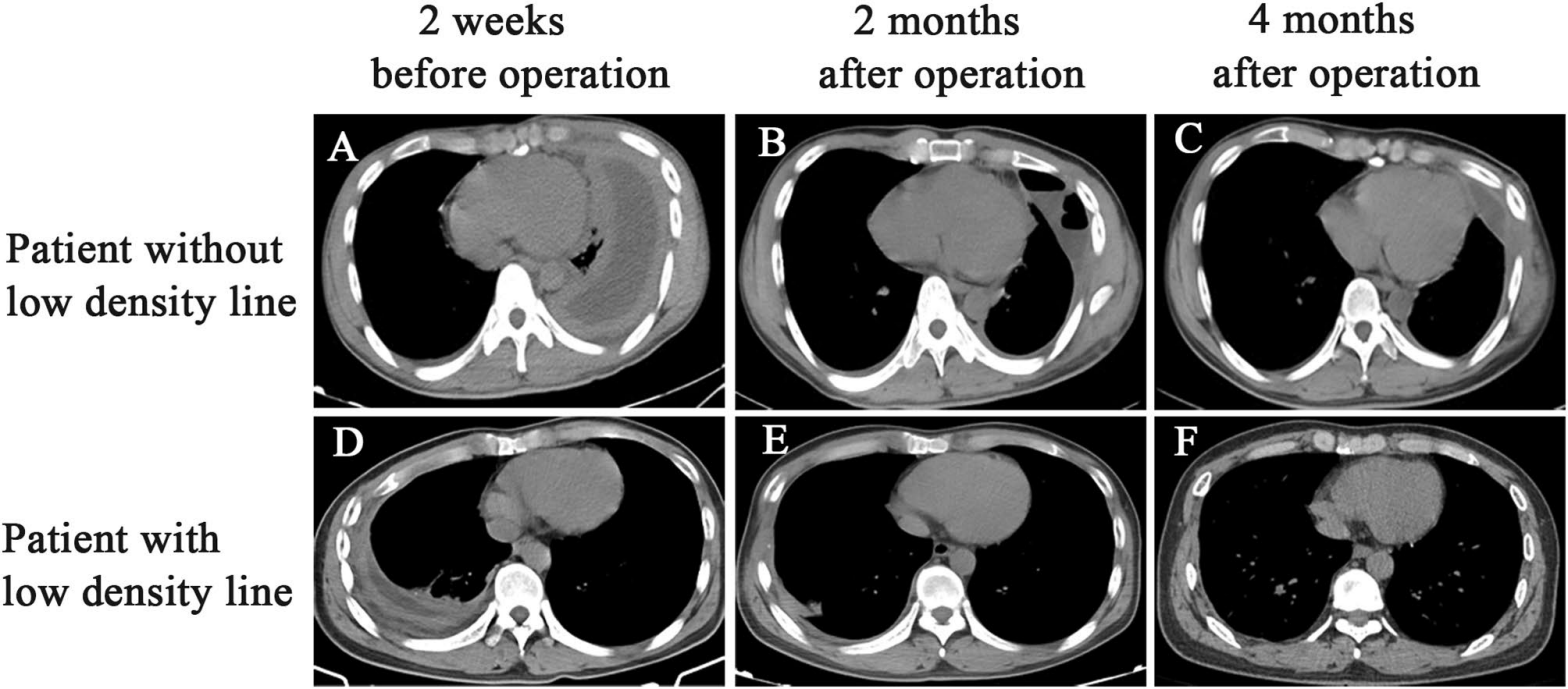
Recovery of the thorax after surgery. Preoperative CT (A) showed left empyema, fibrous plate formation, and no obvious low-density line. The operation time was 6 h, the intraoperative bleeding volume was 800 mL, the postoperative exudation volume was 910 mL, and the duration of drainage was 29 days. (B,C) Are 2 months and 4 months after the operation, respectively. Preoperative CT (D) showed right empyema, fibrous plate formation, and a low-density line. The operation lasted 4.5 h, the intraoperative bleeding volume was 300 mL, the postoperative exudation volume was 580 mL, and the duration of drainage was 7 days. (E,F) were 2 months and 4 months after the operation, respectively.
